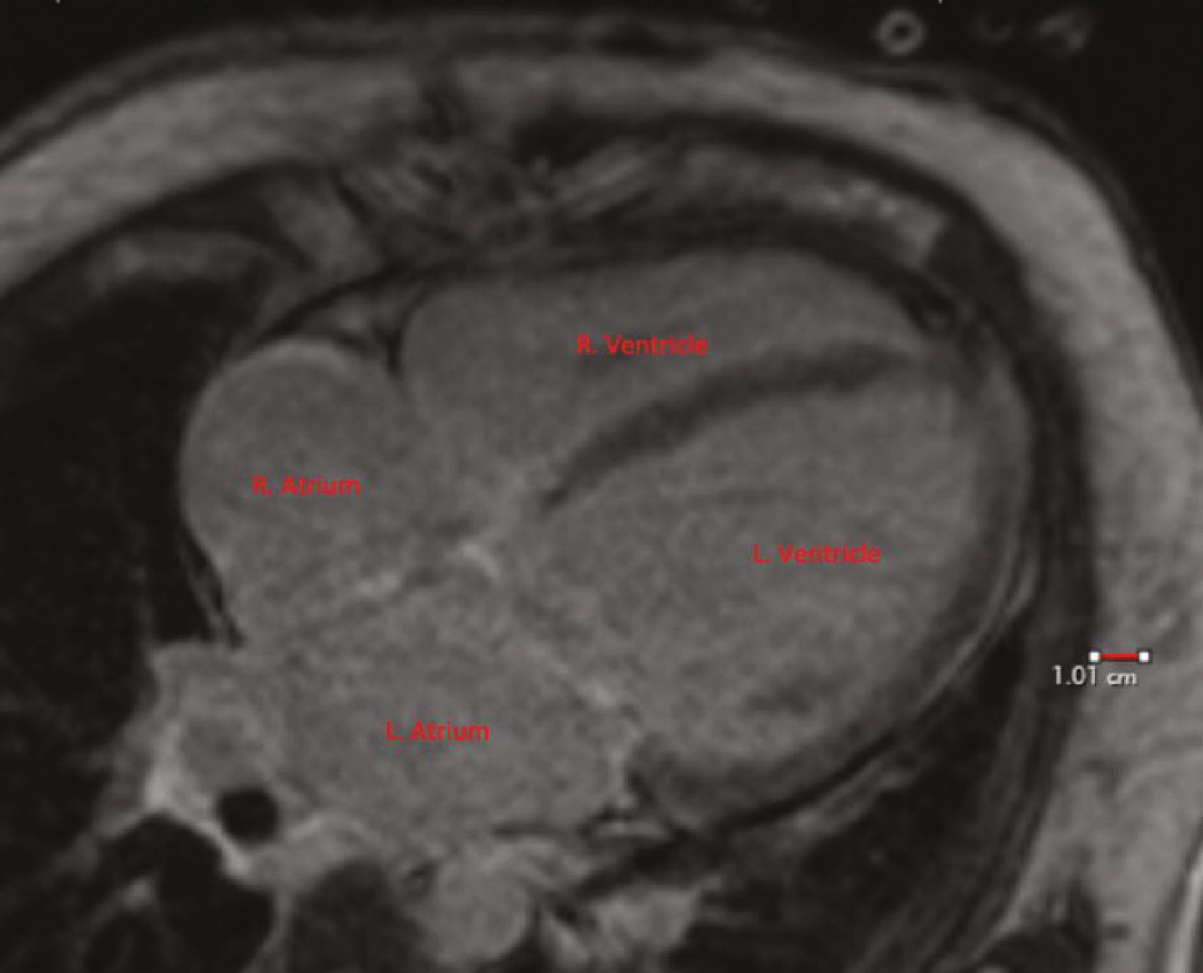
Cardiac MRI showing normal enhancement on delayed gadolinium-enhanced imaging. The red bar is a standard reference ruler. Markers are placed to indicate the anatomical locations of the ventricles and atria.
